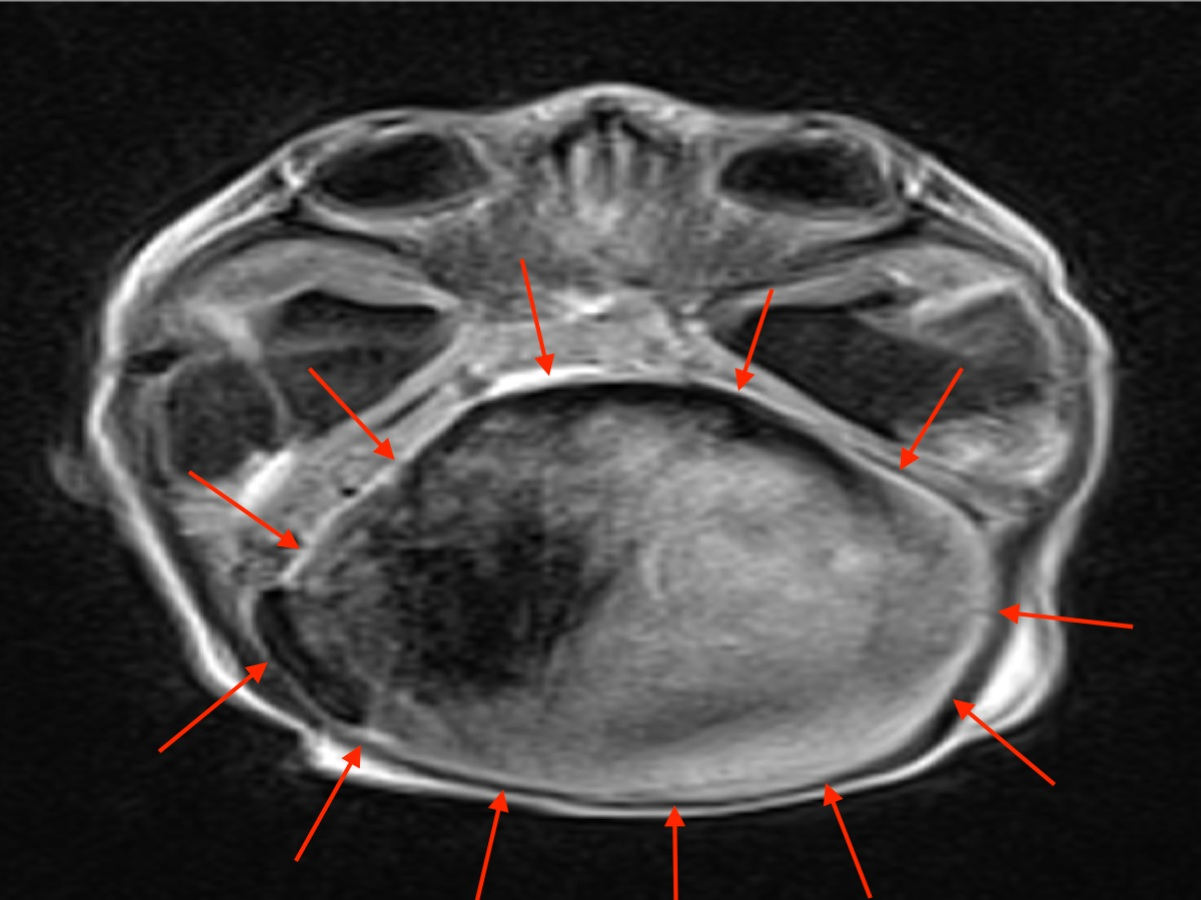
Axial T1-weighted MRI of the patient above with a large VGAM (red arrows) MRI: magnetic resonance imaging; VGAM: vein of Galen aneurysmal malformation.
